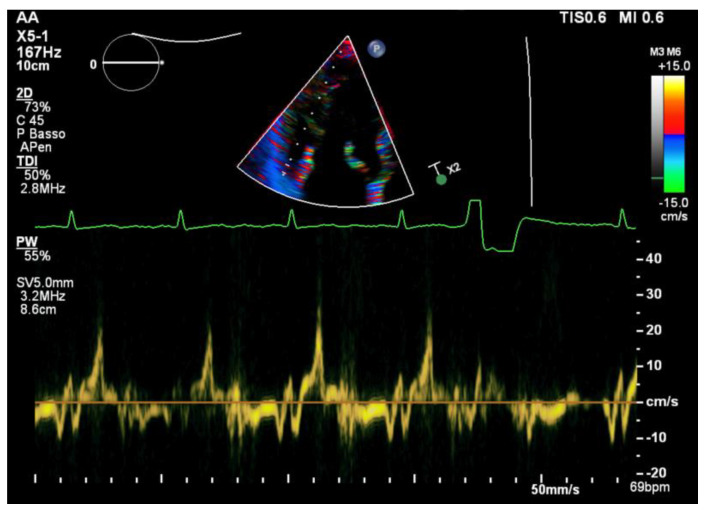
A pulsed wave tissue Doppler signal on the basal inferolateral wall from the same patient of Figure 1 (aMVP group). Note the spiked high velocity signal (Pickelhaube sign).
